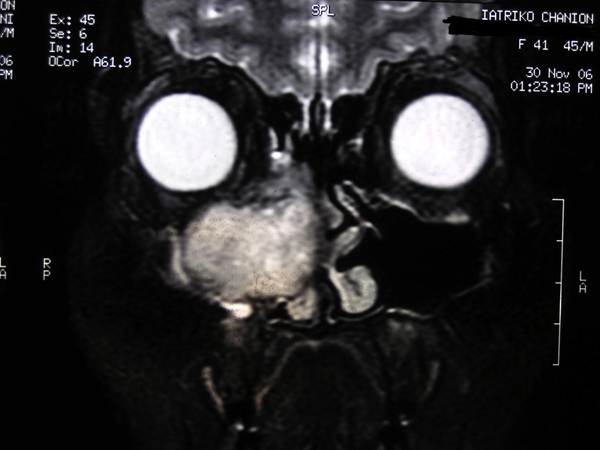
Coronal magnetic resonance imaging of the paranasal sinuses showing a round, bone-expanding lesion in the anterior part of the right maxillary sinus with invasion of the adjacent floor of the orbit, anterior ethmoids and nasal cavity.
